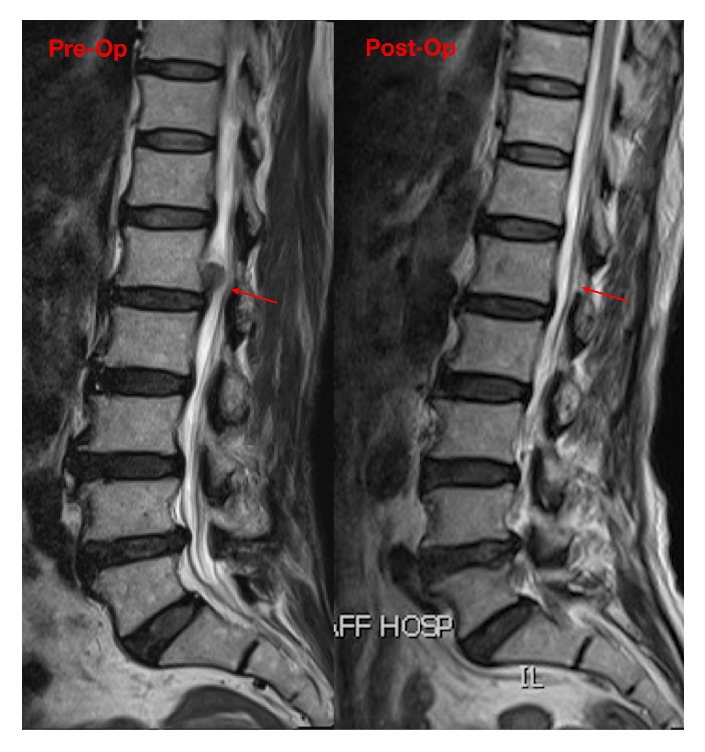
Comparison of magnetic resonance imaging (MRI) findings before and after surgery. Preoperative sagittal plane T2-weighted MRI showing highly upmigrated and sequestrated disc herniation fragments at the L1-L2 level. Postoperative sagittal plane T2-weighted MRI of the same patient demonstrating complete removal of the L1-L2 disc protrusion fragments.
